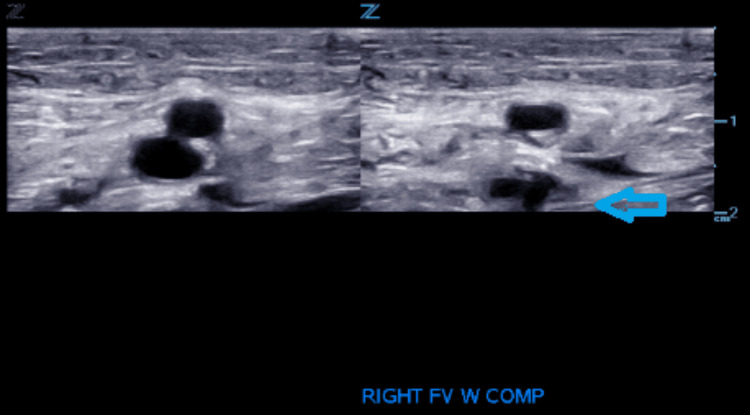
Venous duplex ultrasound: cross-sectional view: right femoral vein non-compressibility indicates thrombus presence.
